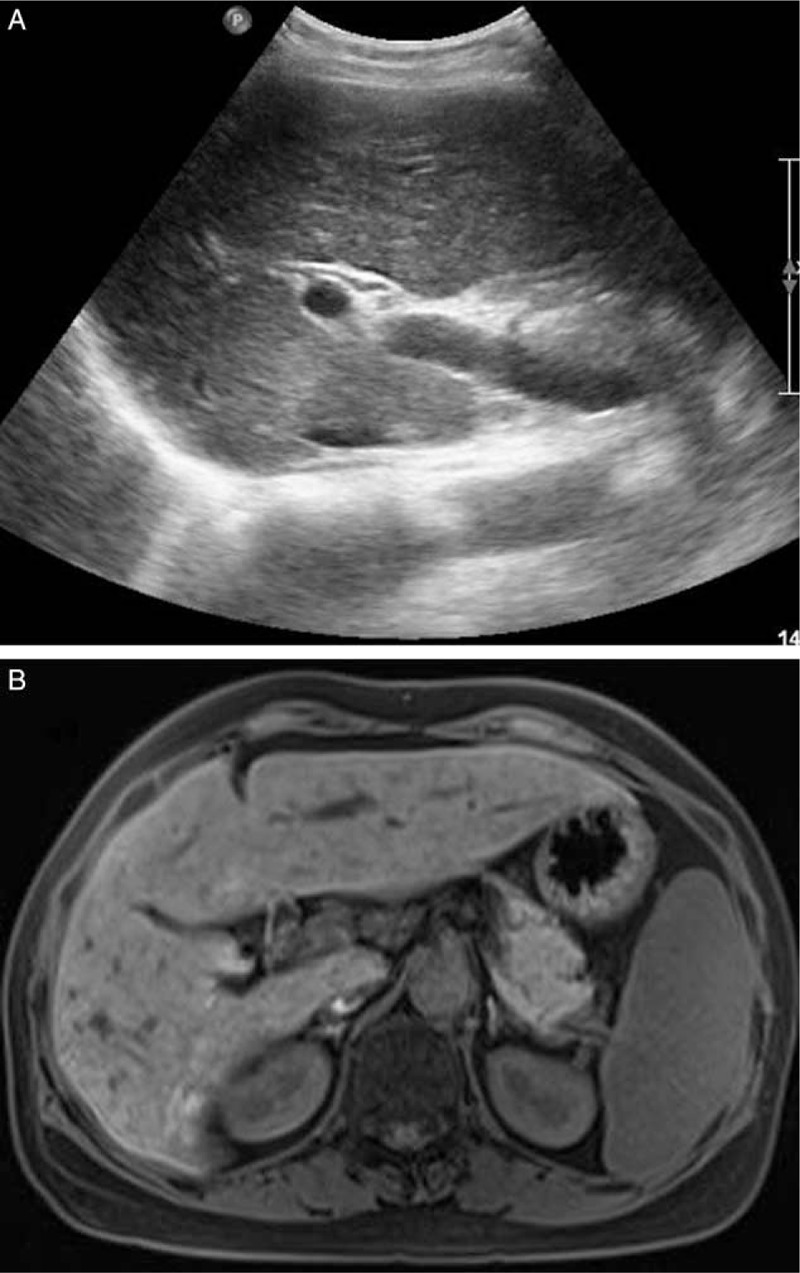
Ultrasound and MRI scan of the patient after the biopsy. The ultrasound exam on POD 1 (A) and MRI on POD 3 (B) show no sign of hematoma and ascites.
